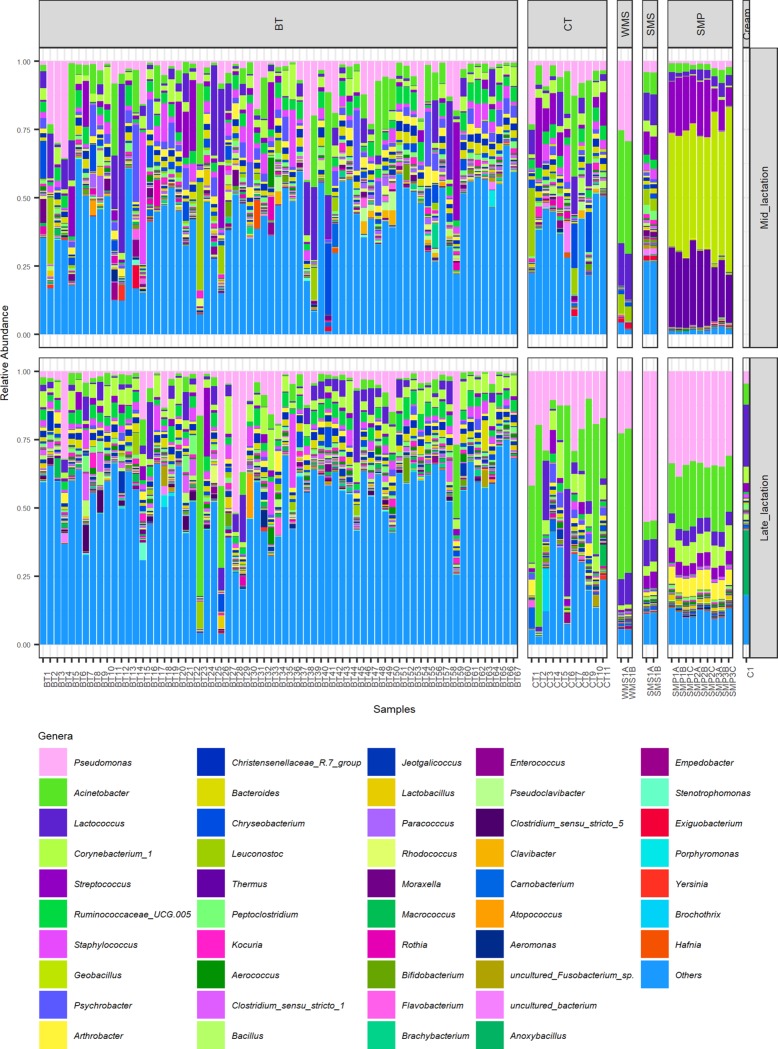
Genera present at a >5% relative abundance in at least one sample. Shown are 16S rRNA gene amplicon sequence data for mid- and late-lactation samples from bulk tanks (BT), collection tankers (CT), whole-milk silos (WMS), skimmed milk silos (SMS), skimmed milk powders (SMP), and cream (late lactation only). Genera shown are present at a >5% relative abundance in at least one sample.
